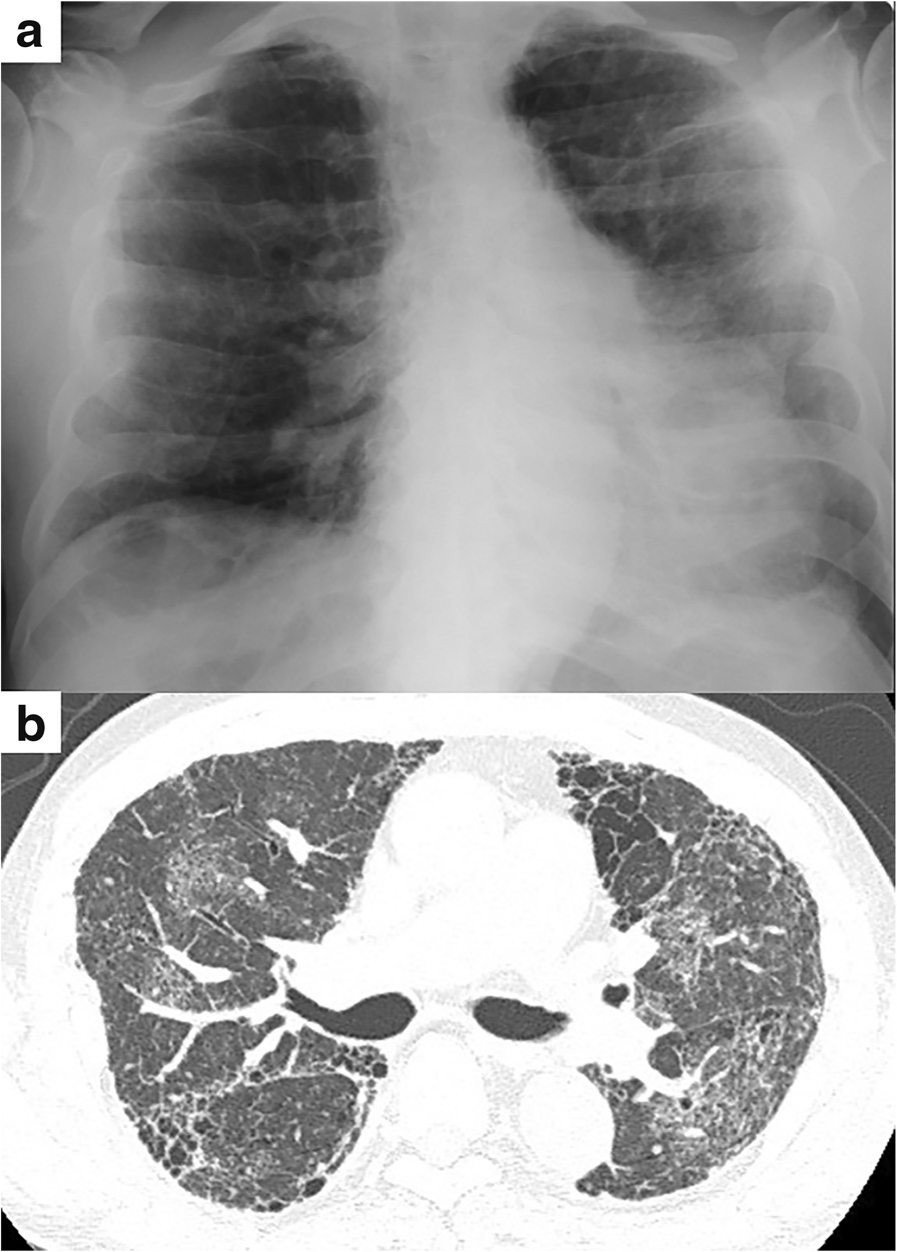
Chest X-ray and high resolution computed tomography at acute exacerbation of idiopathic pulmonary fibrosis onset. Chest X-ray (a) and high resolution computed tomography (b) at the onset of acute exacerbation. On day 68, chest high resolution computed tomography showed newly developed, diffuse, ground glass opacities
